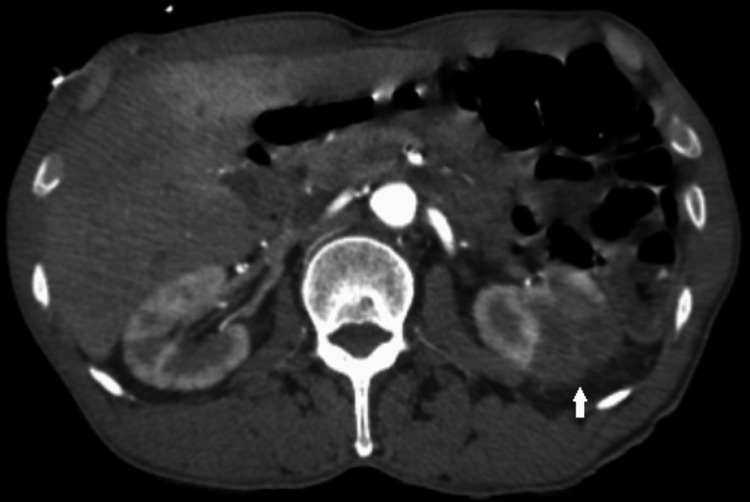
A CTA of the abdomen showed multiple wedge-shaped parenchymal hypodensities in kidneys (arrow). CTA: computed tomography angiography
